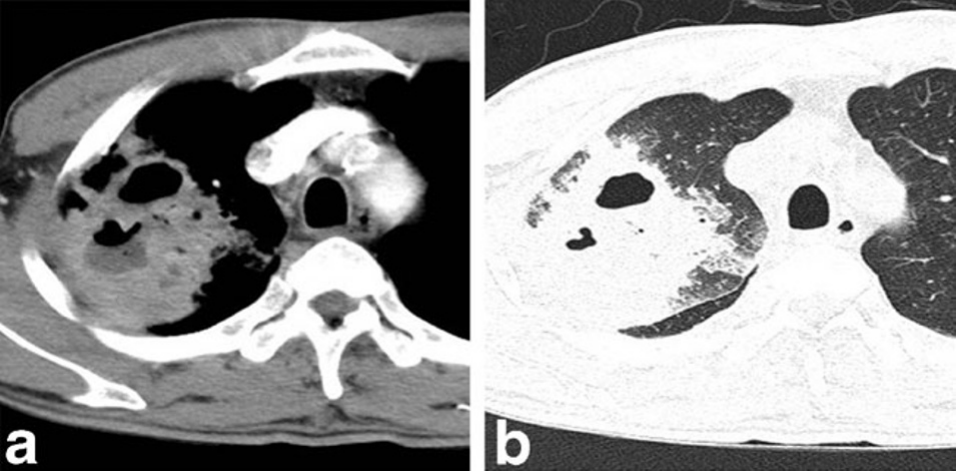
Parenchymal actinomycosis with abscess formation in a 51-year-old man with cough and dyspnoea who had a history of alcoholism. Chest CT scan displayed on a mediastinal setting (a) and thin-section CT (b) show peripheral consolidation with multiple internal cavities, a central low-attenuation area and adjacent pleural thickening in the right upper lobe
